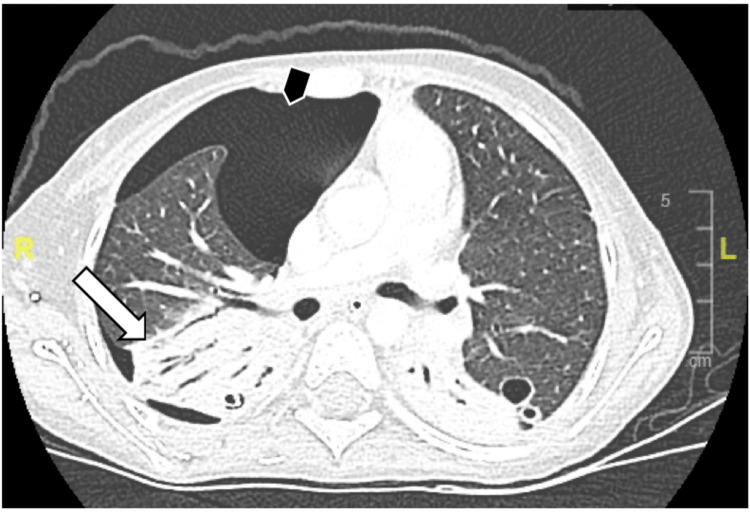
CT chest with contrast in Case 5 demonstrated patchy nodule in the left upper lobe and a small area of ground-glass opacity (white-long arrow), and large right pneumothorax (black-arrow).
